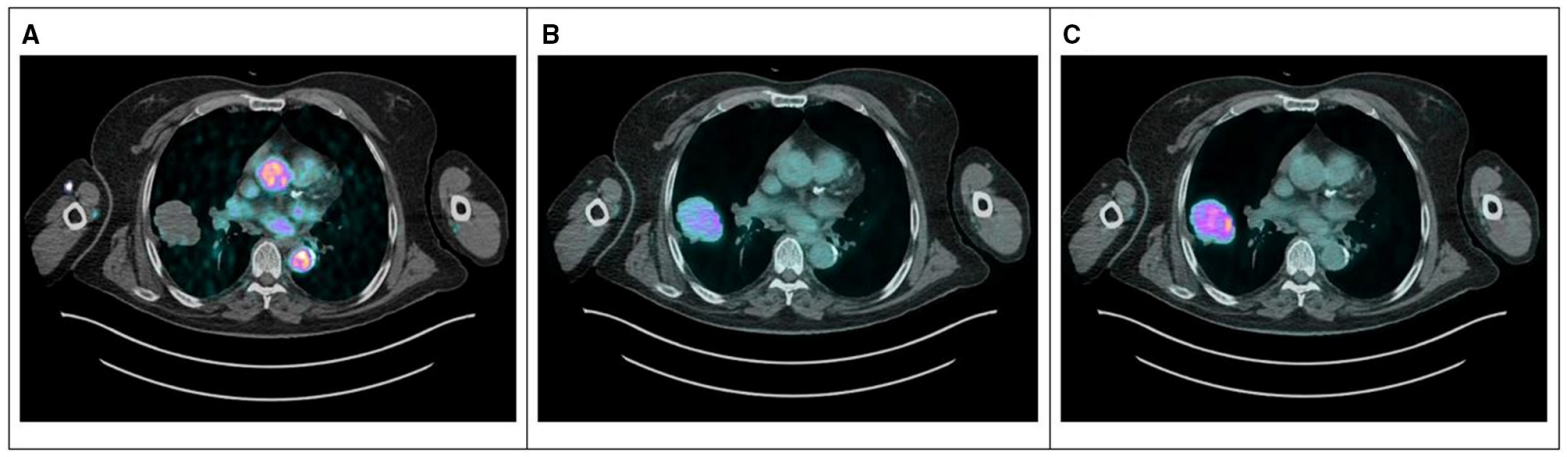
PET/CT images at 2 min (A), 30 min (B), and 60 min (C) after 2-[^18^F]FDG injection for a representative patient (#4) showing the blood pool in the early phase and the increasing uptake in the lesion during the time. 2-[^18^F]FDG, 2-[^18^F]Fluoro-2-deoxy-D-glucose.
